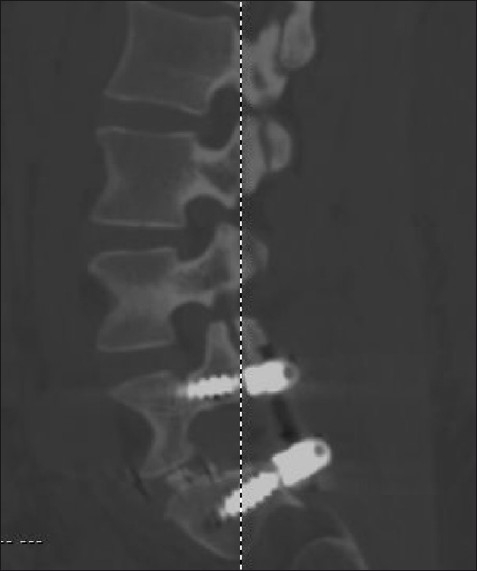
Sagittal reconstruction of the postoperative computed tomography showing the extent of resection of the articular processes and the transpedicular posterior segmental fixation on the right side. A portion of the polyetheretherketone (PEEK) interbody graft is visible
